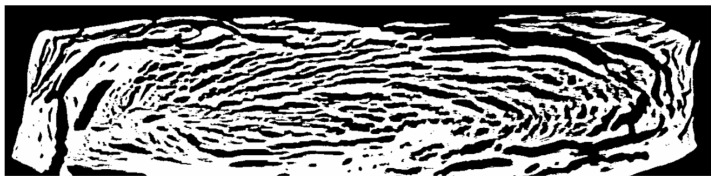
Microstructure of freeze-dried SPI extrudate, obtained with X-ray measurements. Sample was extruded at 50% (*w/w*) moisture content and material temperature of 124 °C before entering the cooling die. Front view is displayed. Flow direction is directed out of the image plane towards the reader.
